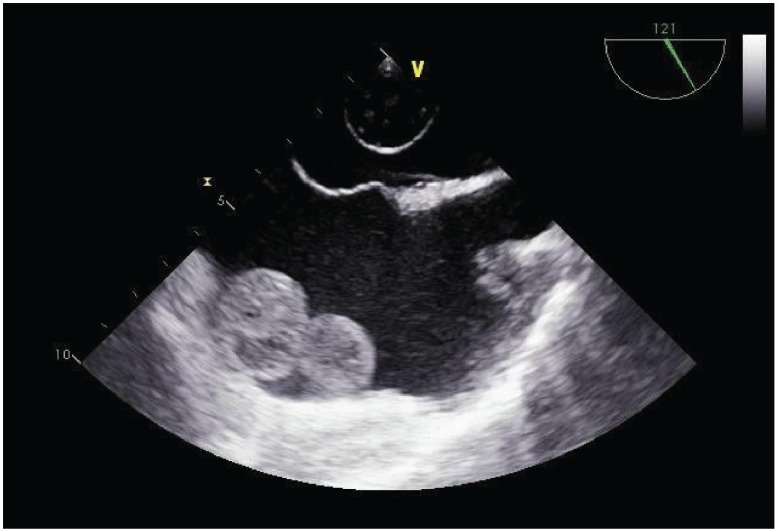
Transesophageal echocardiogram of the mass both in the right and left atria.
